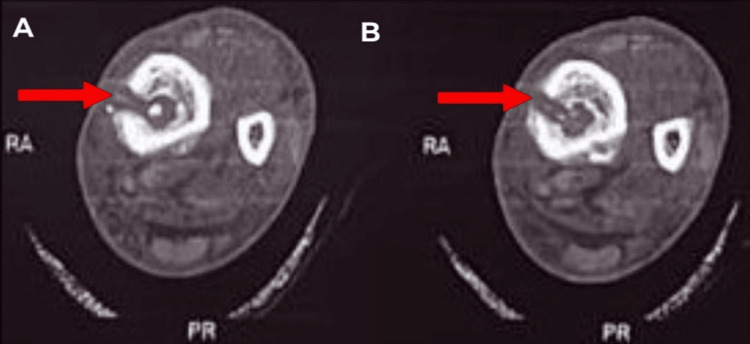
A-B) The axial section of the left leg CT scan. Red arrows show a cortical breach in the tibia shaft. CT: computed tomography
